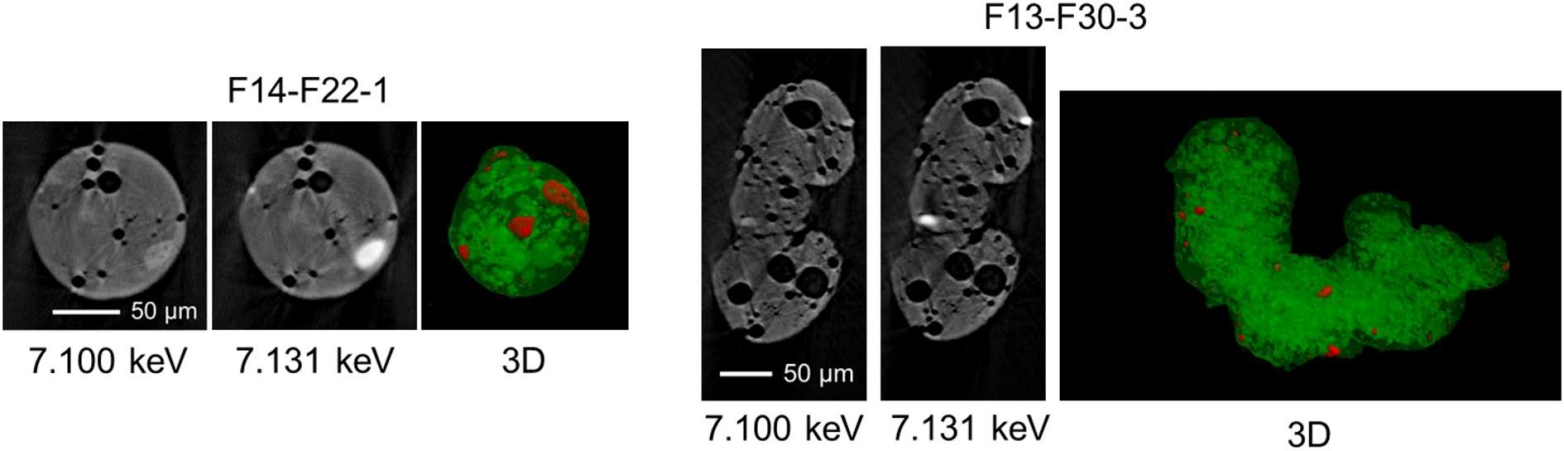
Slice of a µ-X-ray CT image taken at incident X-ray energy below and above Fe K-edge emission for spherical (left) and non-spherical (right) Type-B particles. 3D images are constructed using ImageSurfer (https://www.imagesurfer.org). Many voids exist. The white areas in slice images and the red areas in 3D images indicate Fe-rich parts.
